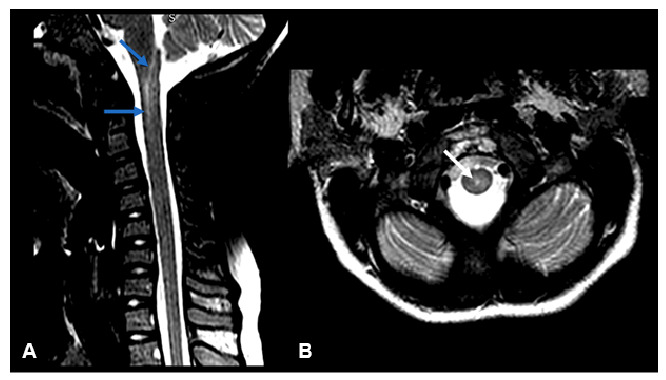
Spine magnetic resonance imaging (MRI) shows a hyperintense signal within the central spinal cord extending from the obex to the C2 level on T2-weighted imaging, visible in both sagittal (arrows in A) and axial (arrow in B) planes, consistent with myelitis. No gadolinium enhancement was observed. Brain MRI findings were normal.
